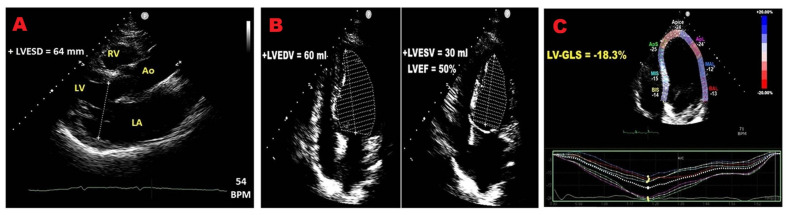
TTE and STE-derived LV indicators of adverse outcome in PMR individuals. (A) Enlarged LV end-systolic diameter. (B) Reduced LVEF. (C) Impaired LV–GLS. GLS, global longitudinal strain; LV, left ventricular; LVEF, left ventricular ejection fraction; LVEDV, left ventricular end-diastolic volume; LVESD, left ventricular end-systolic diameter; LVESV, left ventricular end-systolic volume; PMR, primary mitral regurgitation; STE, speckle tracking echocardiography; TTE, transthoracic echocardiography.
